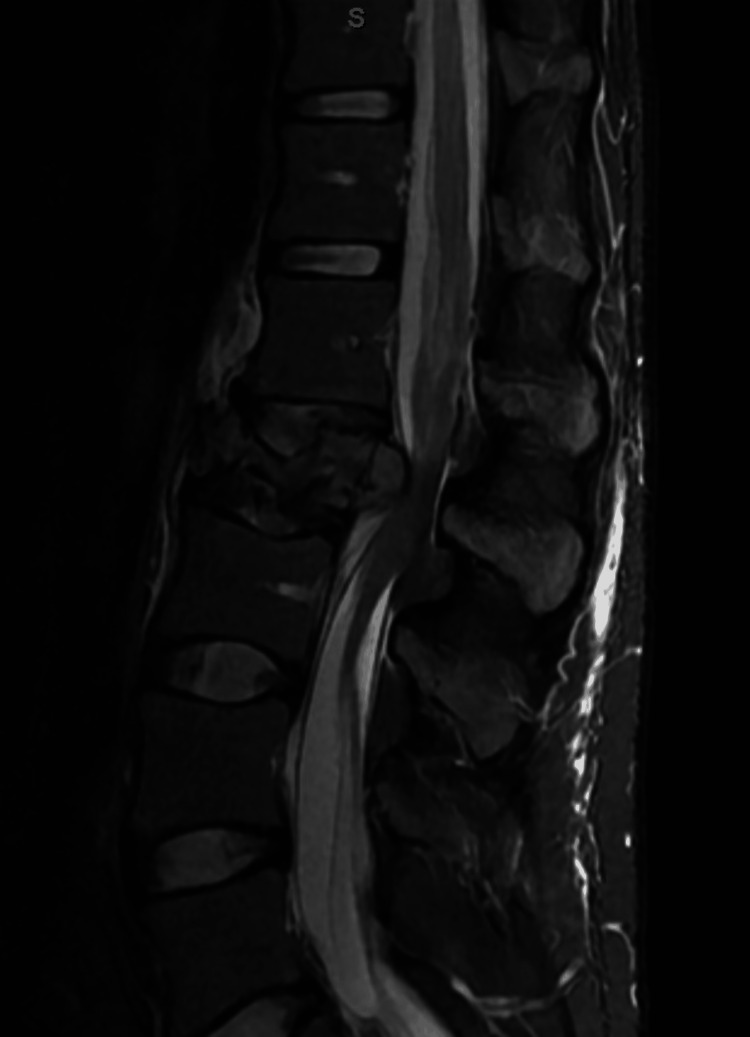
Sagittal section of lumbar spine MRI showing fracture of L2 vertebrae with narrowing of the spinal canal and compression of the cauda equina nerve roots MRI: magnetic resonance imaging, L2: second lumbar vertebrae
